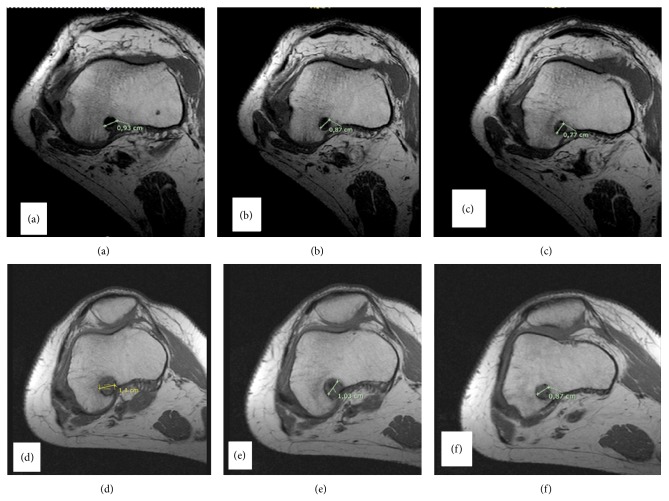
Comparison of femoral tunnel's axial MRI cuts performed on the same patient 1 and 12 months following ACL reconstruction. (a) Proximal 1 month, (b) middistance 1 month, (c) distal 1 month, (d) proximal 12 months, (e) middistance 12 months, and (f) distal 12 months.
